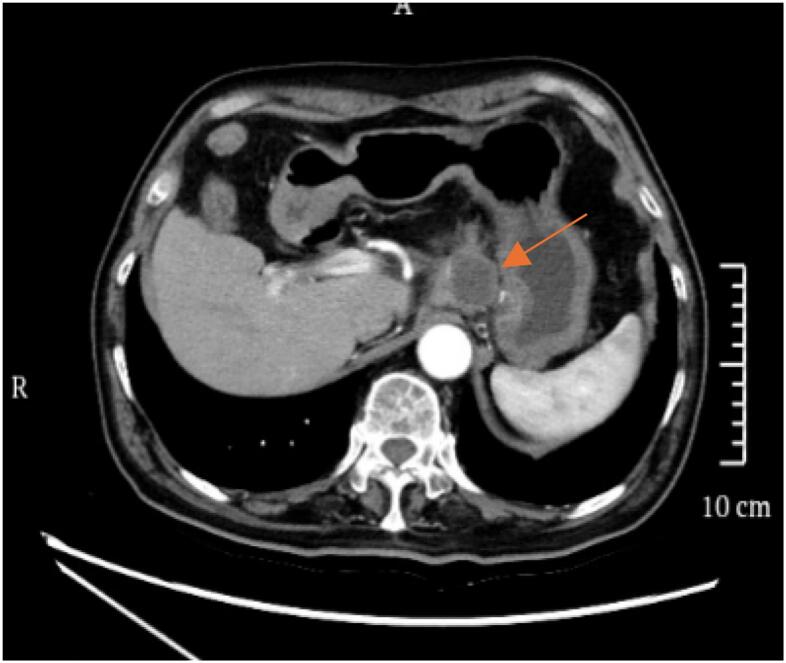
Computed tomography image showing a retroperitoneal mass near the lesser curvature of the stomach, measuring 32 mm × 31 mm.
